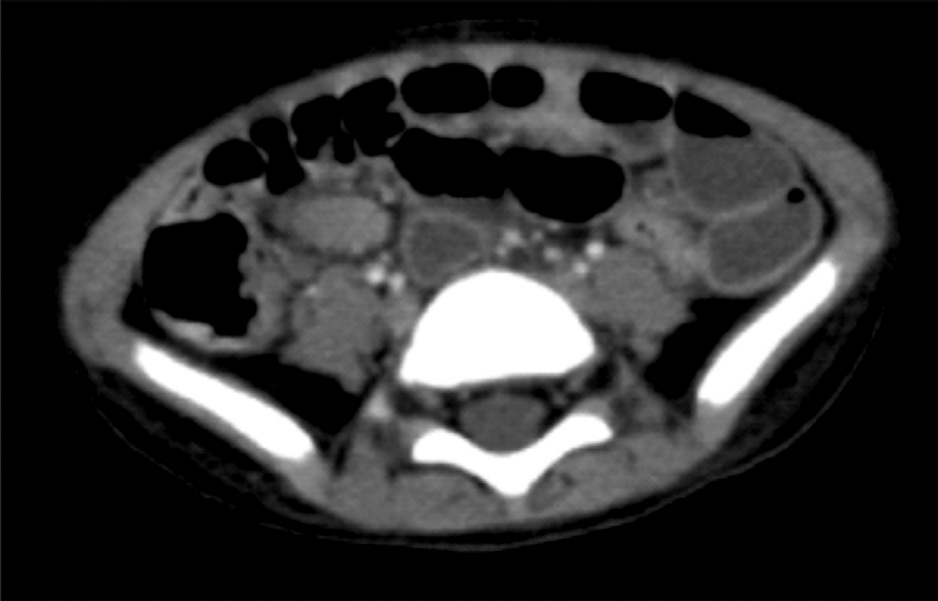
Abdominal CT-Scan with contrast showing multiple mesenteric lymphadenopathies accompanied by liver enlargement and minimal ascites.
